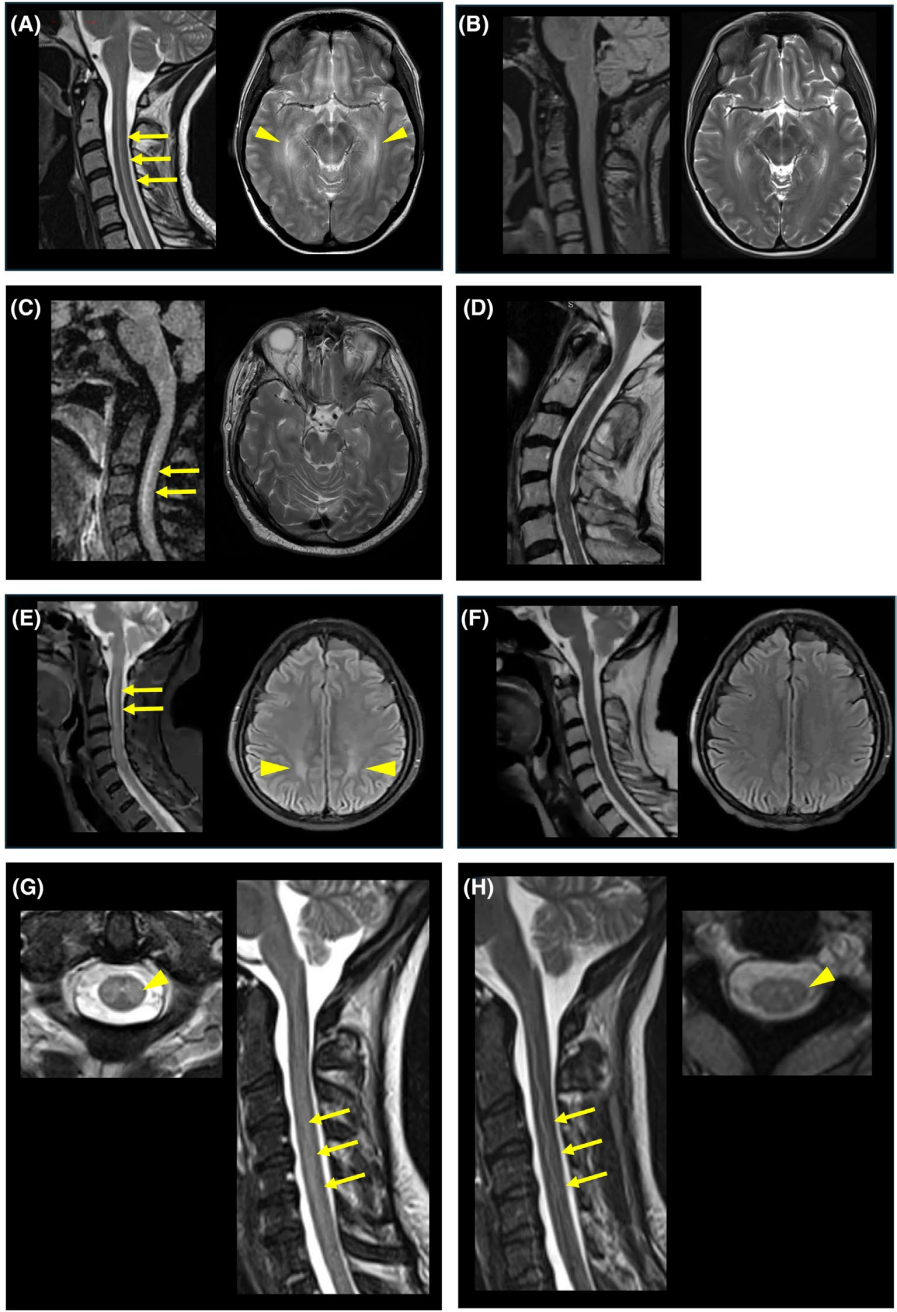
Magnetic resonance images for the four patients. Patient 1: T2‐weighted sagittal image of the cervical spine and axial image of the brain from day 7 (panel A) demonstrating widespread hyperintensity in the corticospinal tracts in the spine (arrows) and brain (arrow heads). Panel (B) shows T2/FLAIR image of the sagittal spine and T2‐weighted axial image of the brain from day 17, demonstrating slight improvement. Patient 2: T2/FLAIR sagittal image of the cervical spine and T2‐weighted axial image of the brain from day 6 (panel C) demonstrating longitudinally extensive signal change in the spine (arrows) and patchy hyperintensity in the brain. Panel (D) shows a sagittal T2‐weighted image of the cervical spine from day 21 demonstrating resolution of previous signal hyperintensity. Patient 3: T2‐weighted/FLAIR sagittal image of the cervical spine and axial image of the brain from day 7 (panel E) showing extensive, symmetrical signal hyperintensity throughout both posterior frontal, parietal and medial temporal lobes (arrow heads), with longitudinally extensive central cord hyperintensity in the cervical spine (arrows). Signal change extended throughout the length of the spine (not shown). The signal changes have completely resolved by day 24 (panel F). Patient 4: T2‐weighted axial and sagittal images of the cervical spine on day 7 (panel G) demonstrate hyperintensity within the white matter tracts (arrows and arrowhead). Repeat imaging on day 12 (panel H) shows marked regression, with some residual signal change. FLAIR, fluid‐attenuated inversion recovery.
